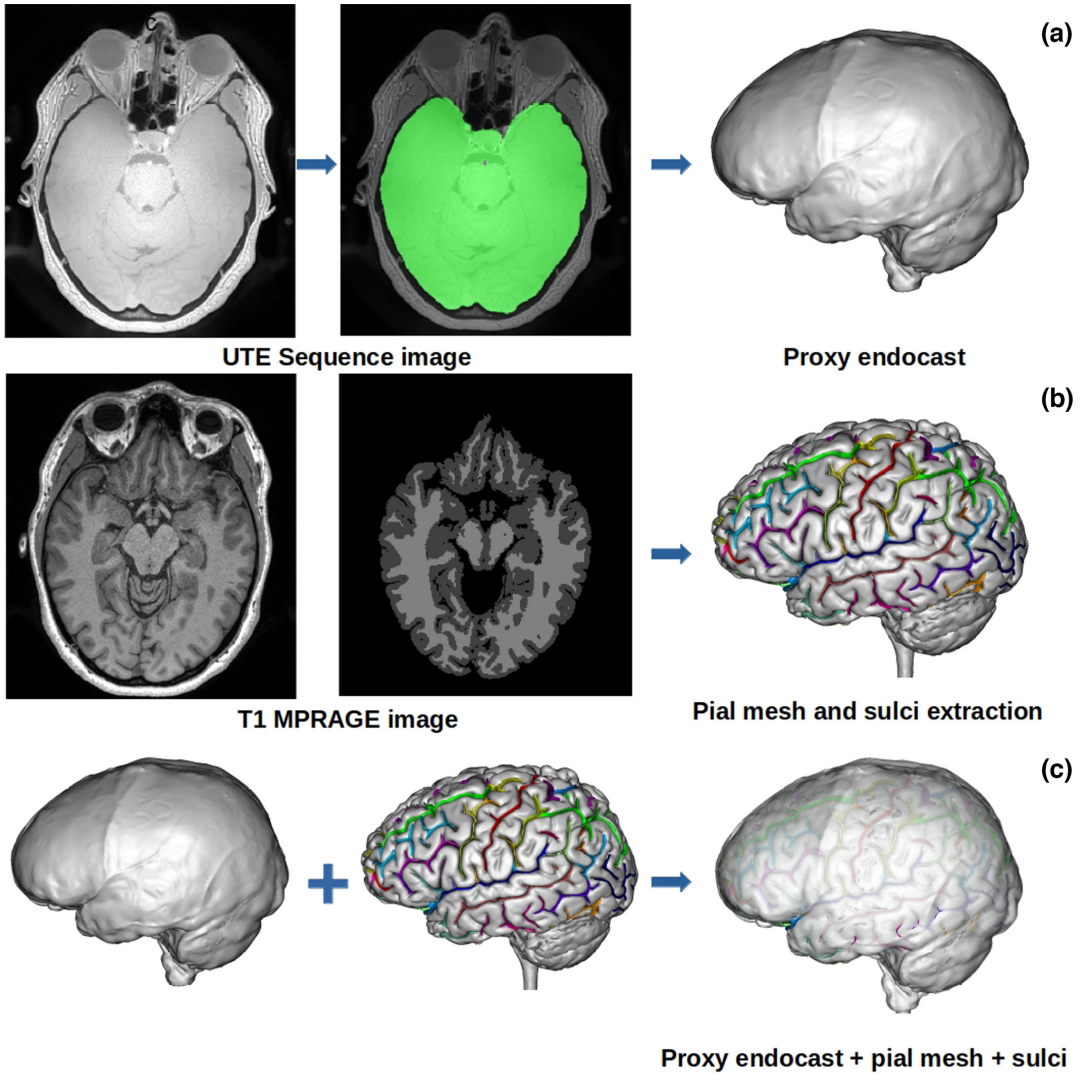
Brain structures and meshes used for manual labelling of sulci. (a) Proxy endocast obtained from an MRI UTE image. (b) Structures (pial mesh and automatic sulci extraction) obtained from the brain segmentation using a T_1_‐MPRAGE image. (c) Superimposition of the proxy endocast, the pial mesh and the automatically extracted sulci used for the evaluation of manual sulcal identification.
